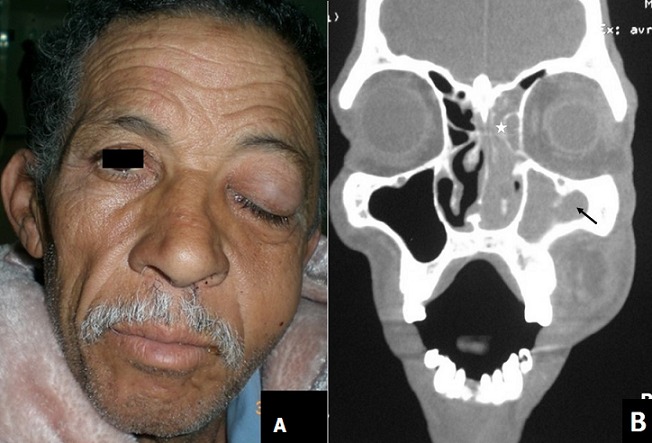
(A) clinical examination at the second day of hospitalization found a ptosis of the left eye associated with edema of the left cheek; (B) coronal computed tomography scan showed soft tissue opacification of the left maxillary (arrow) and ethmoid sinuses (star) without orbital involvement
